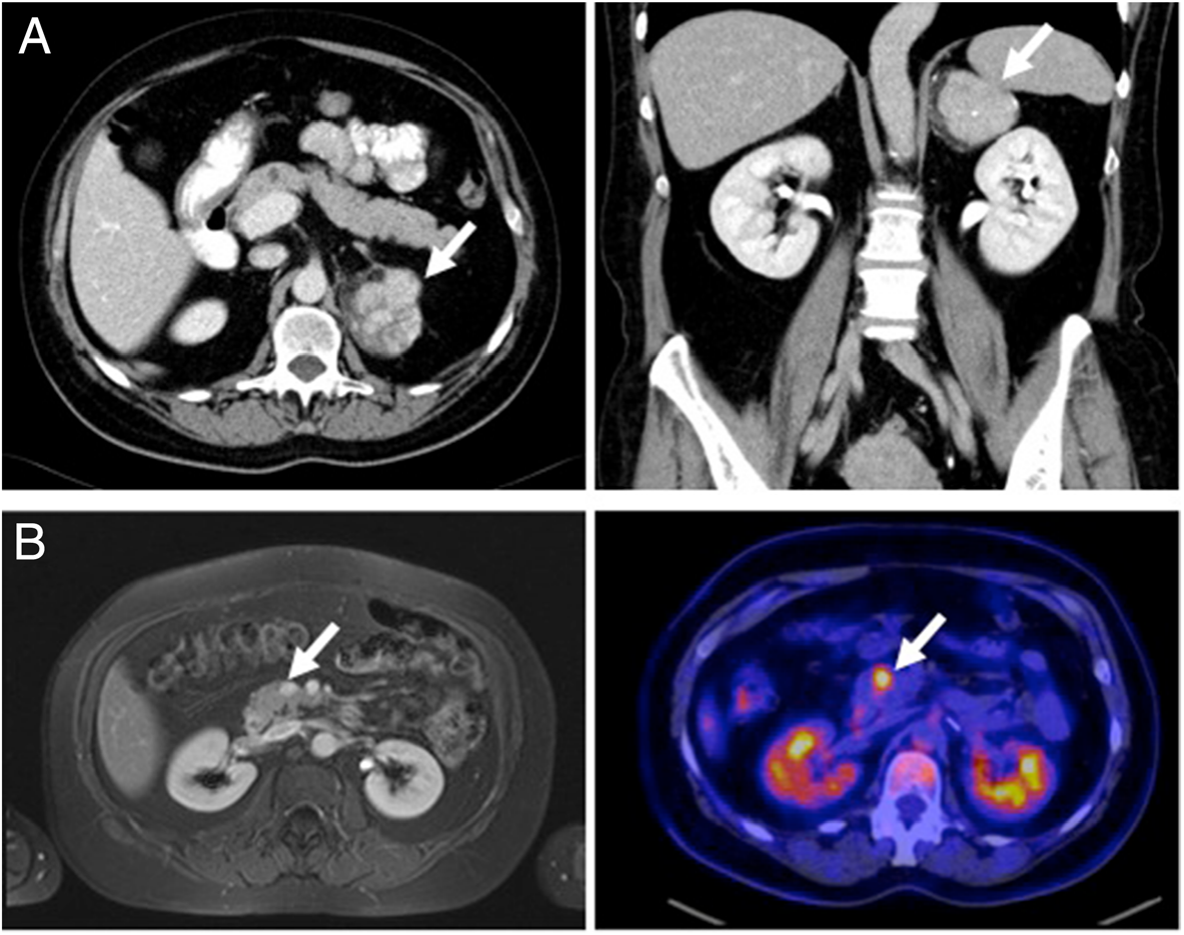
Imaging of the primary adrenocortical carcinoma (a) and the solitary metachronous metastasis inside the pancreatic head (b) by MRT and FDG-PET/CT, respectively
